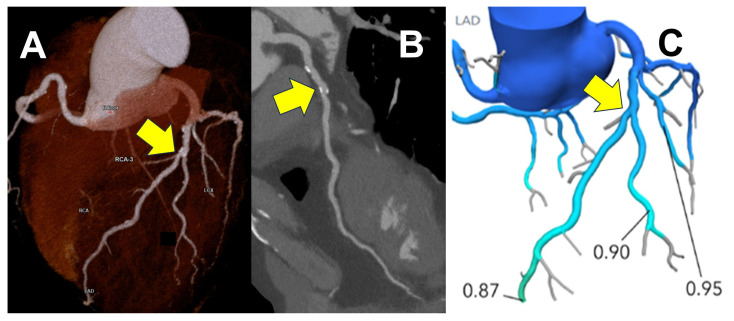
FFR-CT enables detailed noninvasive coronary artery assessment in cases where invasive testing is undesirable. This is a coronary CT image of a patient before bentall procedure. Findings suggestive of moderate stenosis were suspected in the left anterior descending artery (LAD), necessitating a more detailed preoperative ischemic evaluation (A,B) (yellow arrows). Since invasive coronary angiography was risky, FFR-CT was performed (C). It revealed no functional ischemia in the coronary arteries, including the LAD (yellow arrow), allowing avoidance of invasive testing.
